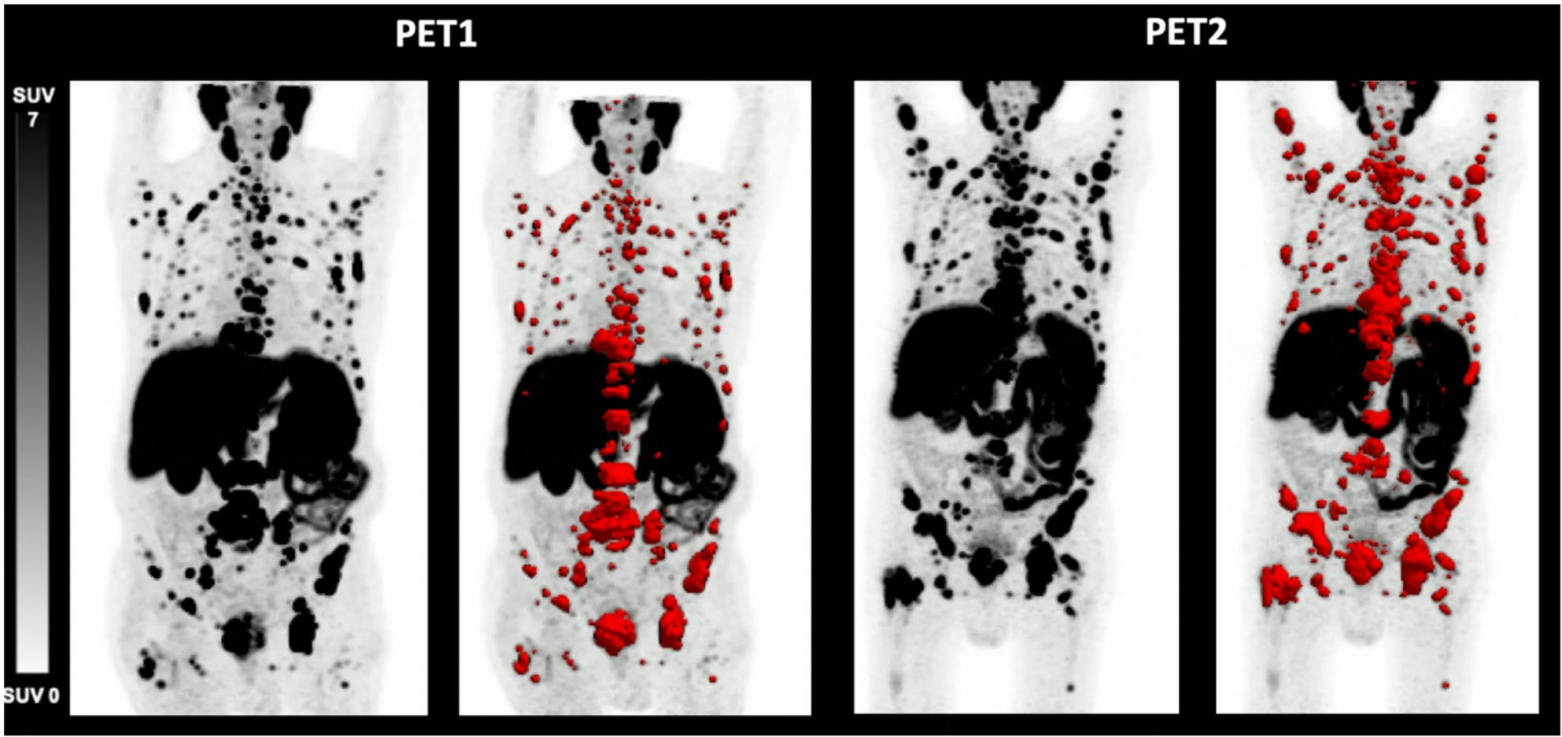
[^18^F]PSMA-1007 PET/CT derived maximum intensity projection (MIP) of a 75-year-old patient with metastatic prostate cancer before (PET1) and after (PET2) initiation of apalutamide therapy (time difference 1.60 months). TTV (+ 47.1%) increased, whereas SUV_max_ (− 34.6%) and SUV_mean_ (− 28.5%) decreased, PSA level decreased from 289.00 ng/mL to 55.00 ng/mL. This patient was rated as SD according to RECIP 1.0
